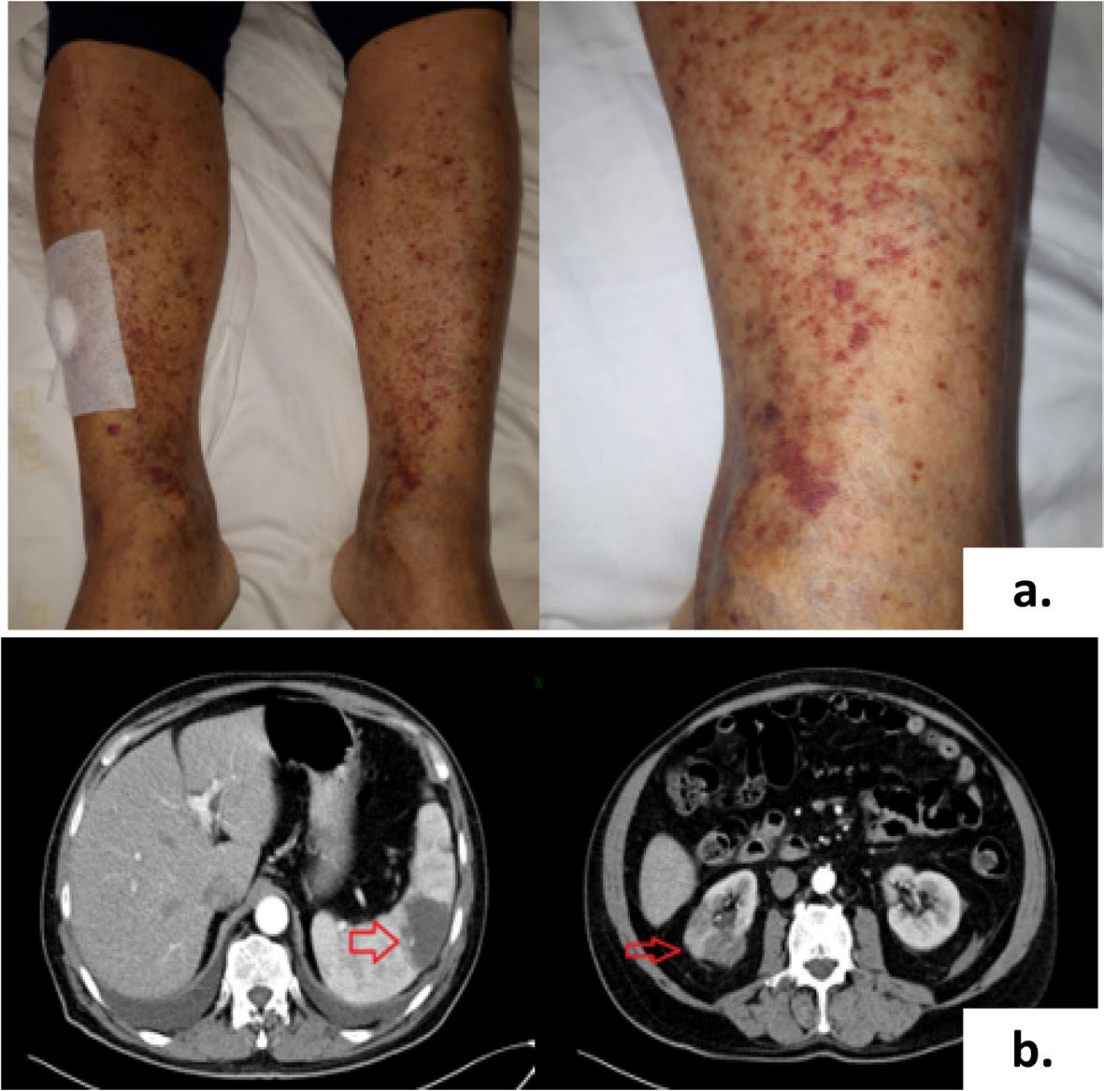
a. Purpura-shaped pigmentation in lower limbs bilaterally. b. CT-scan of the abdomen and pelvis showing infarction areas presumably due to septic emboli in spleen and right kidney
